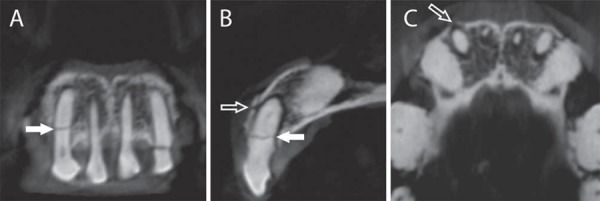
A: CBCT coronal image of the right lateral incisor, showing root fracture (filled arrow); B: CBCT sagittal image of the right lateral incisor, depicting root fracture (filled arrow) and alveolar fracture (outlined arrow); C: CBCT axial image of the right lateral incisor, showing alveolar fracture (outlined arrow)
